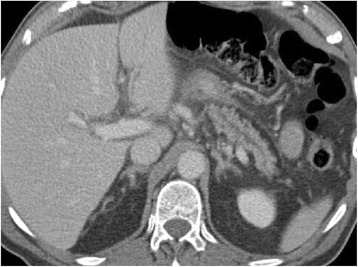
CT of the abdomen (portalvenous phase) at the initial presentation shows a dilated pancreatic duct without evidence of an obstructive process or cholelithiasis
